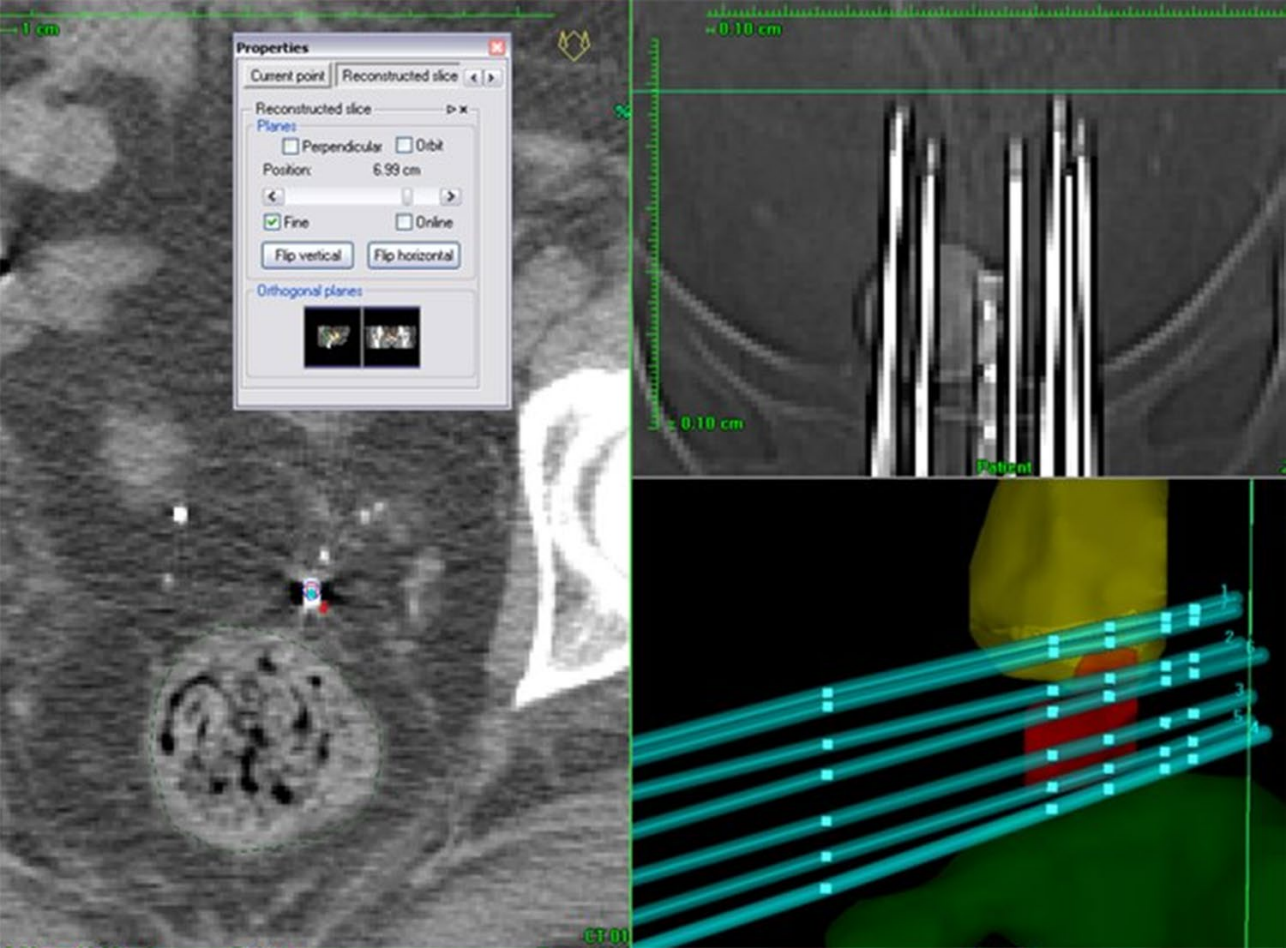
Use of scout view to determine the needle tip on CT images
